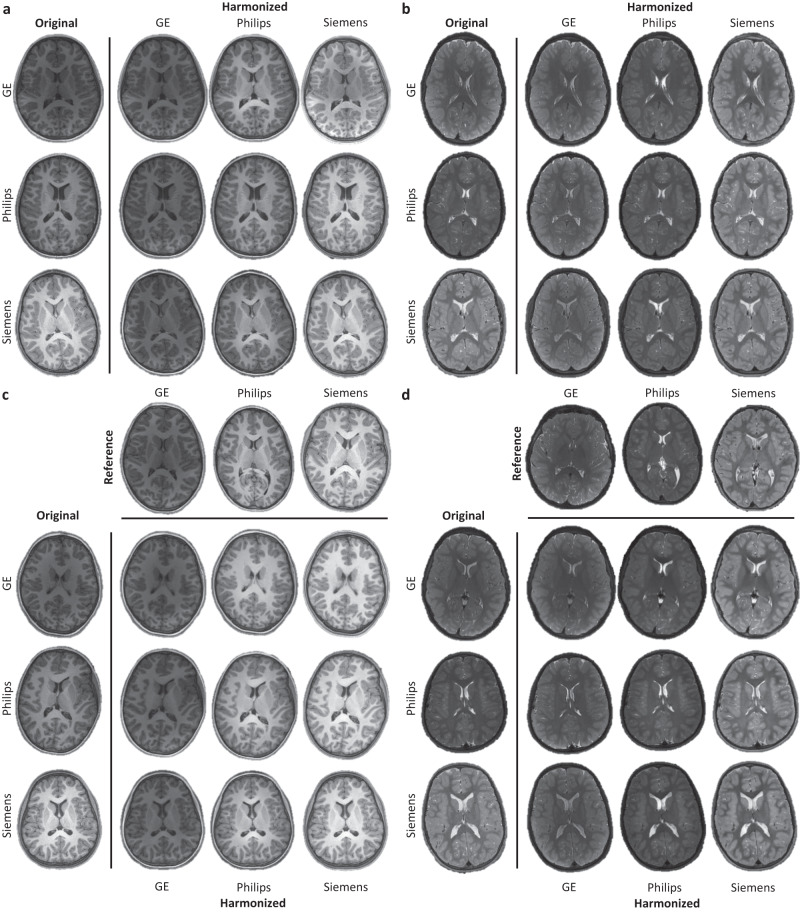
Harmonization of T1- and T2-weighted Images. Site-specific harmonization of a T1-weighted images and b T2-weighted images. Reference-specific harmonization of c T1-weighted images and d T2-weighted images. The original images are shown in the first column and the harmonized images are shown for General Electric (GE), Philips, and Siemens respectively, in the second to fourth columns.
